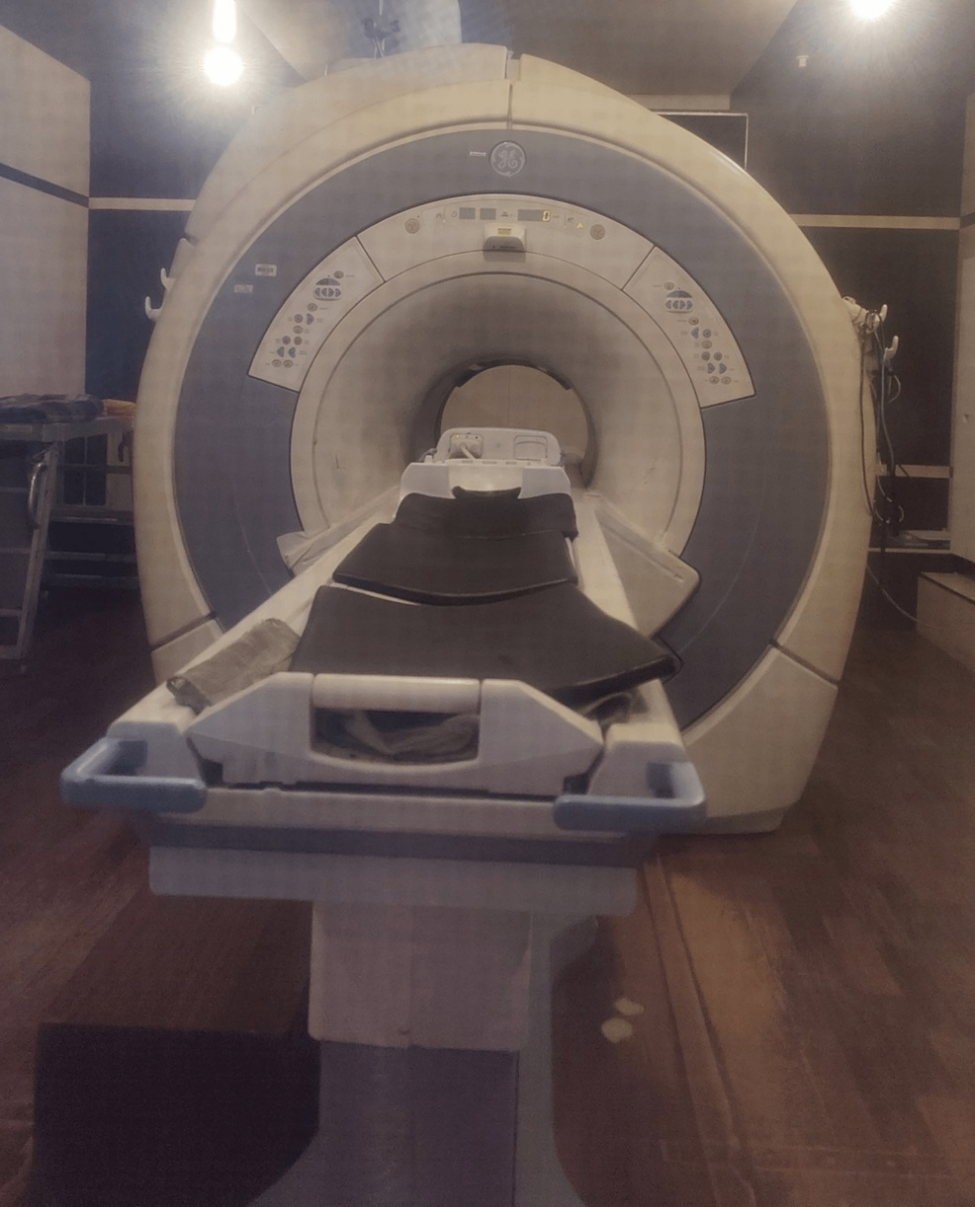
MRI machine Image credit: Harsh Lahoti
